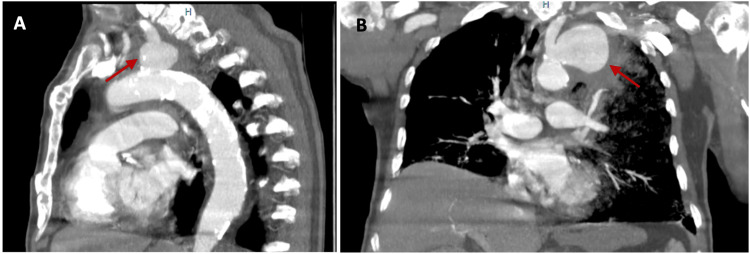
Chest CT angiography: (A) sagittal and (B) coronal images showing the saccular aneurysm at the origin of the subclavian artery (red arrows). CT, computed tomography
